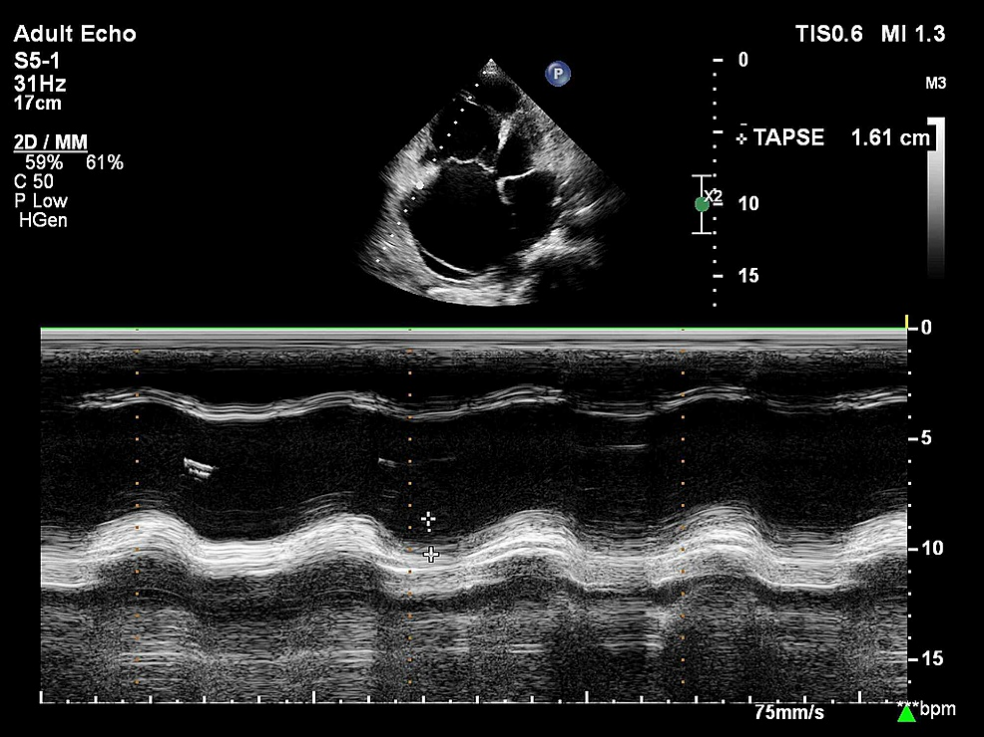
Motion mode echocardiography section demonstrating tricuspid annular plane systolic excursion (TAPSE)
